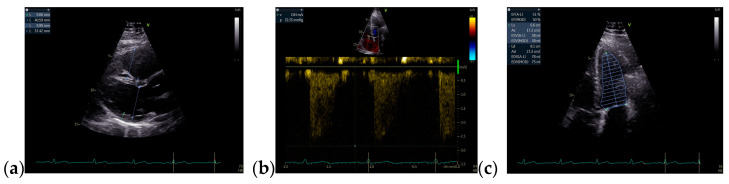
TTE on admission: (a) Parasternal long axis view with enlarged diameter of RV; (b) Apical 4-chamber view, tricuspid continuous wave Doppler flow, with a maximum RV-RA gradient of 32.33 mmHg; (c) Apical 4-chamber view, EF = 51% (Simpson’s method); Abbreviations: TTE—transthoracic echocardiography; RV—right ventricle; RA—right atrium; EF—ejection fraction.
